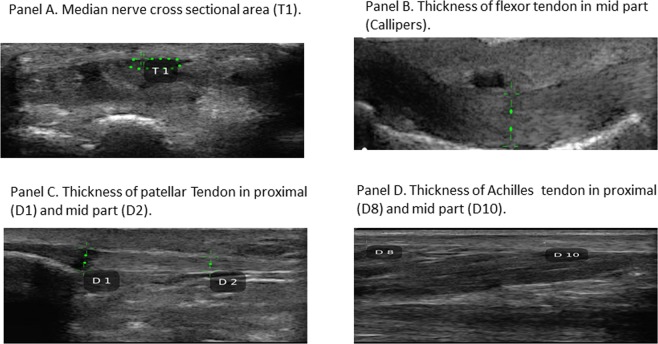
An overview divided in Panels (A–D) of the measurements of the median nerve cross sectional area and of tendon thickness using the handheld sonographic machine.
